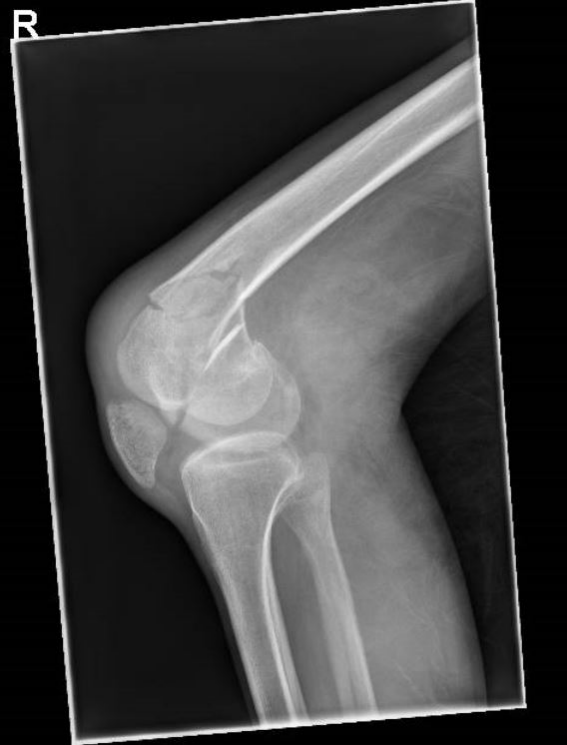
Use of plain radiographs to determine fracture configuration, size of bony comminution and bone stock. Lateral Radiograph.
